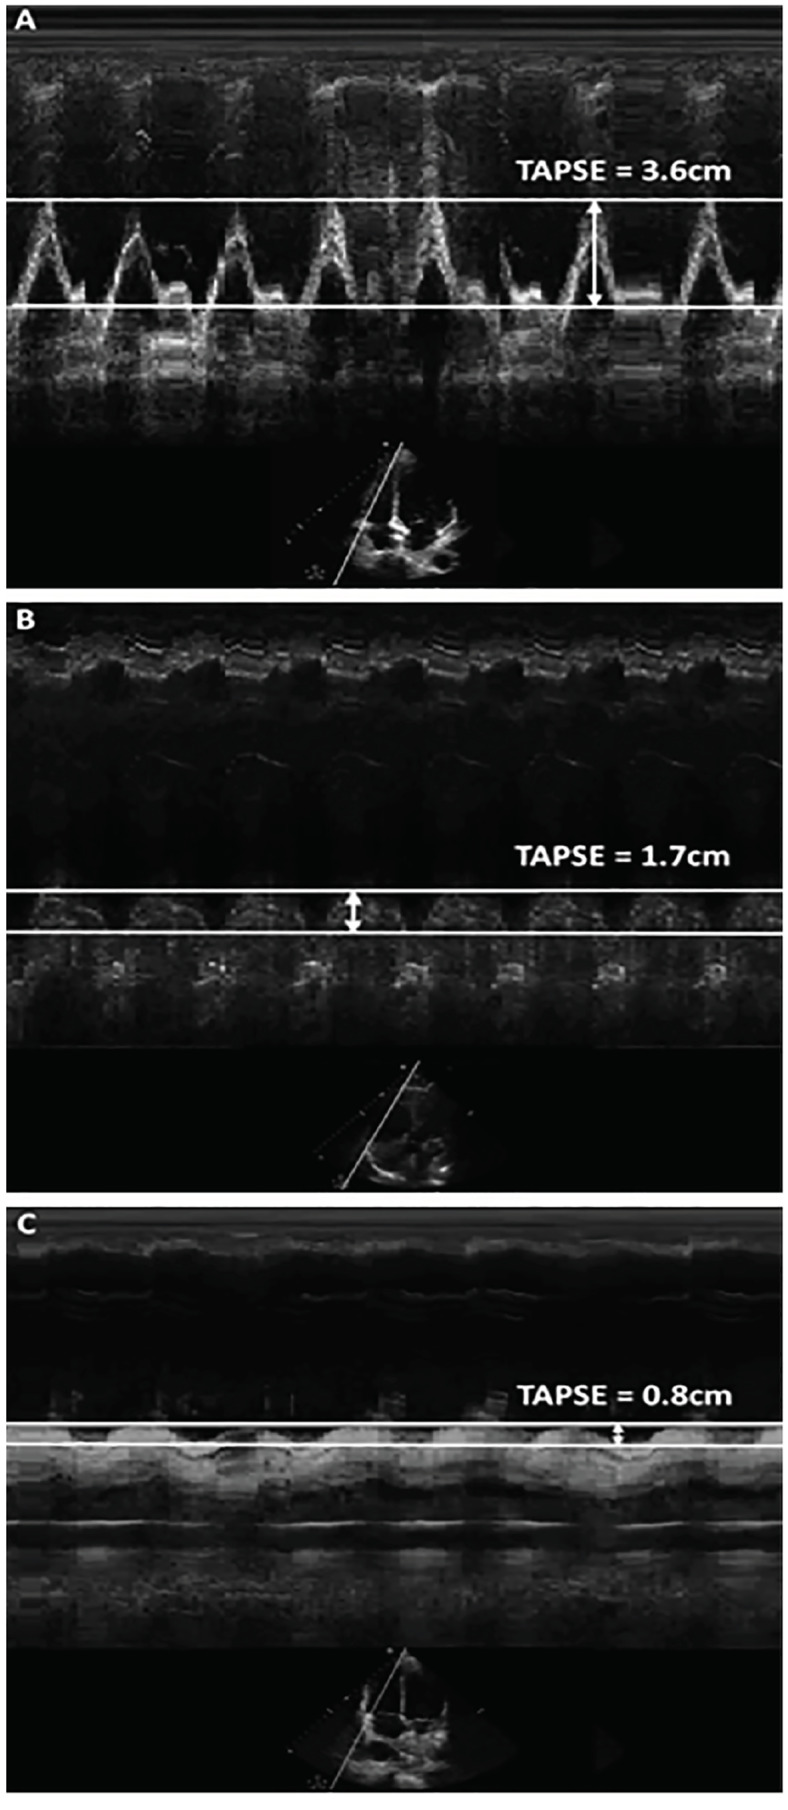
The measurement of tricuspid annular plane systolic excursion (TAPSE) from apical 4-chamber ultrasound clips by using the M.mode.ify open source software in normal (A), borderline (B), and abnormal (C) TAPSE. This technique was applied to determine the reference standard TAPSE measurement for each clip. M-mode images were not shown to the participants. *TAPSE*, tricuspid annular plane systolic excursion; *cm*, centimeter.
